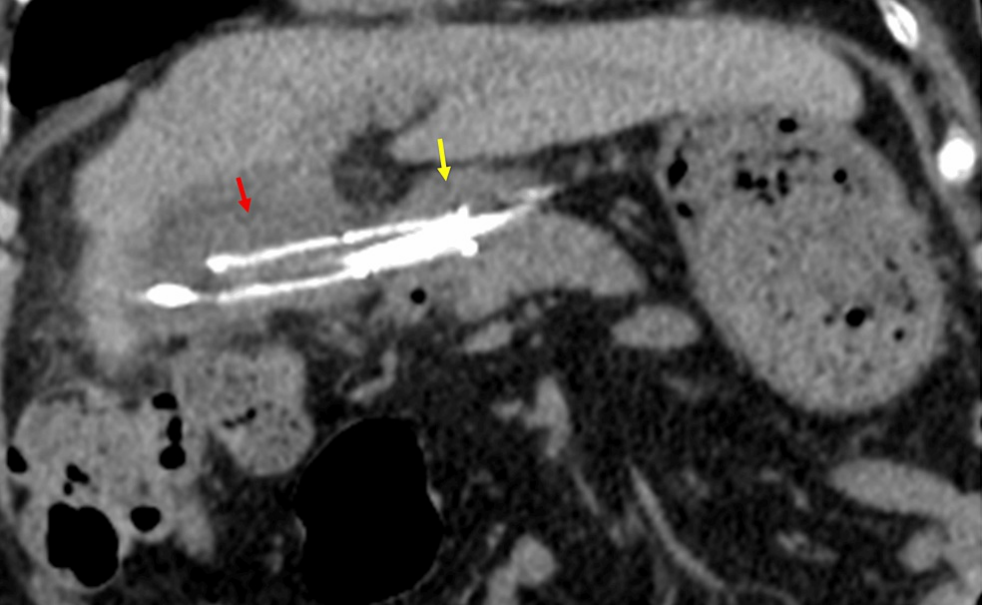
Computed tomography of the coronal view on post-EUS-GBD day seven The stent was located from the gallbladder (red arrow) to the duodenum (yellow arrow). EUS-GBD: endoscopic ultrasound-guided gallbladder drainage
